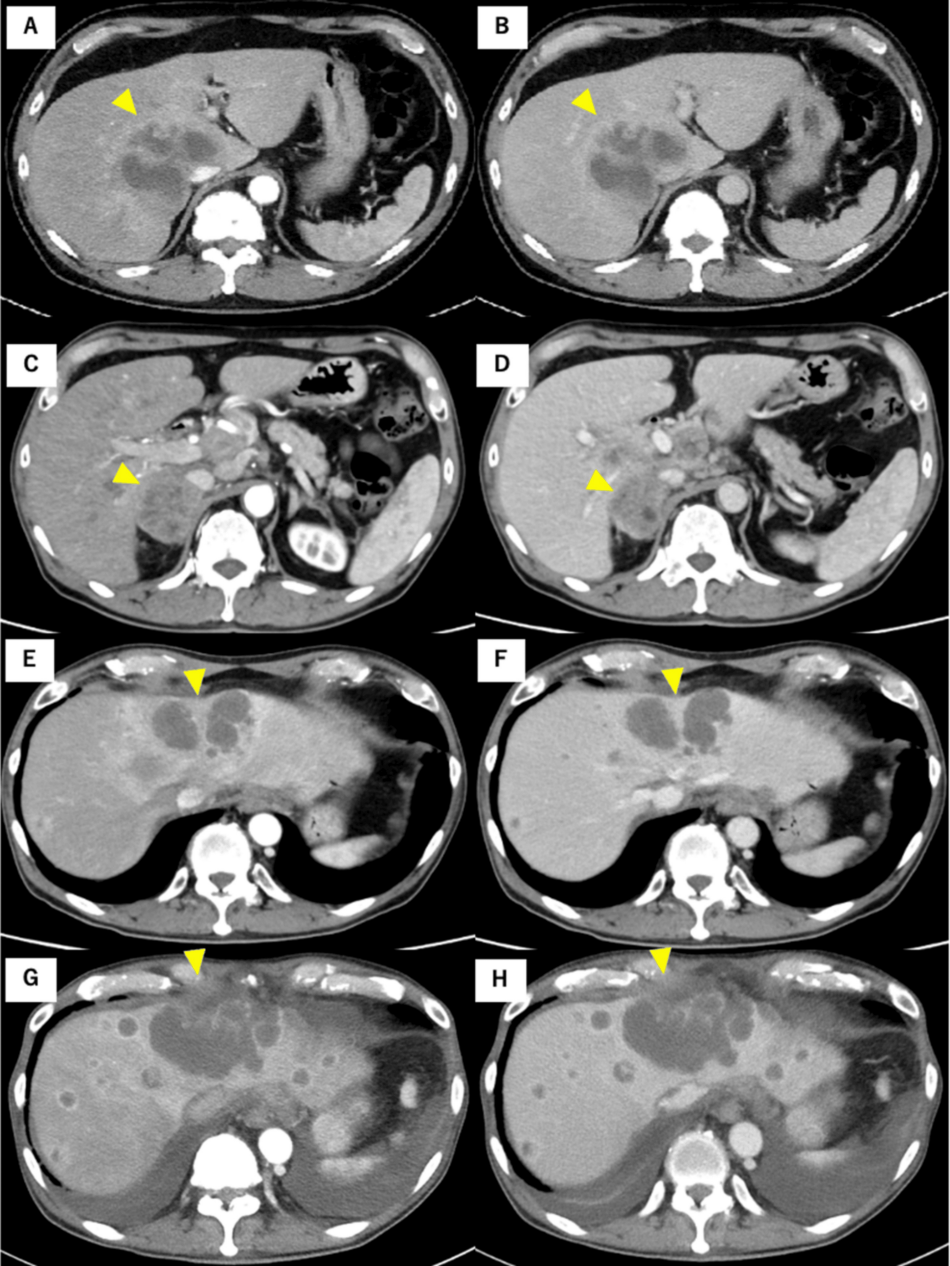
Computed tomography findings. (A) and (B) Before treatment: (A) Arterial phase and (B) portal phase showing a 77-mm low-density mass with contrast enhancement, localized to the caudate lobe and segment 7. A slight contrast effect is noted in the portal phase.
